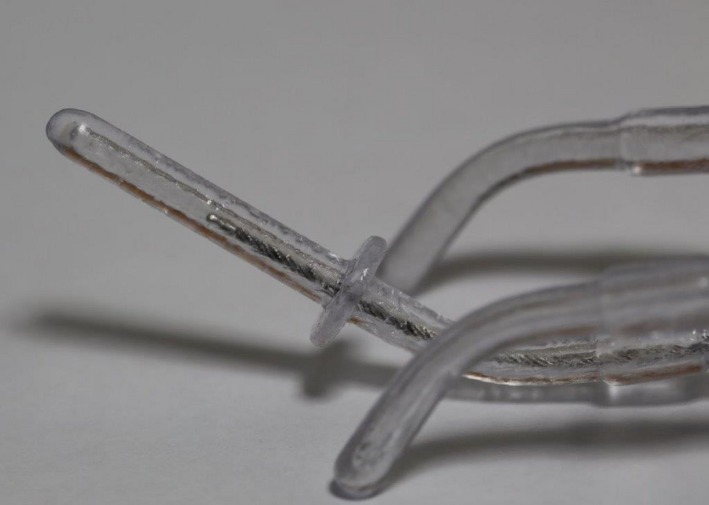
Appearance of a transparent applicator model of the Fletcher CT/MR applicator.
